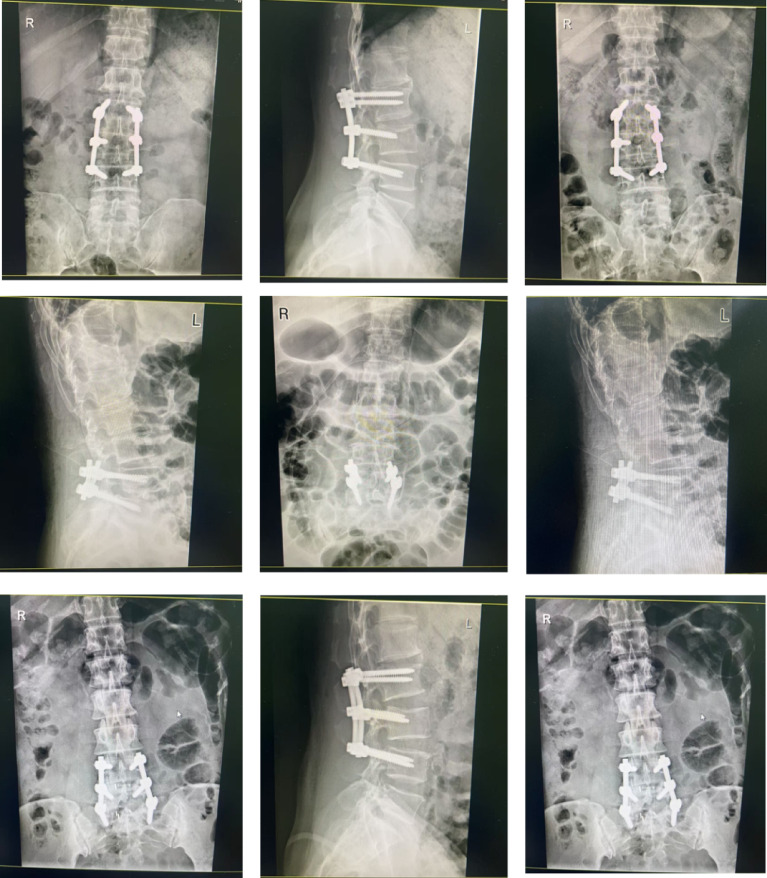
Representative lumbar spine radiographs with postoperative instrumentation.
